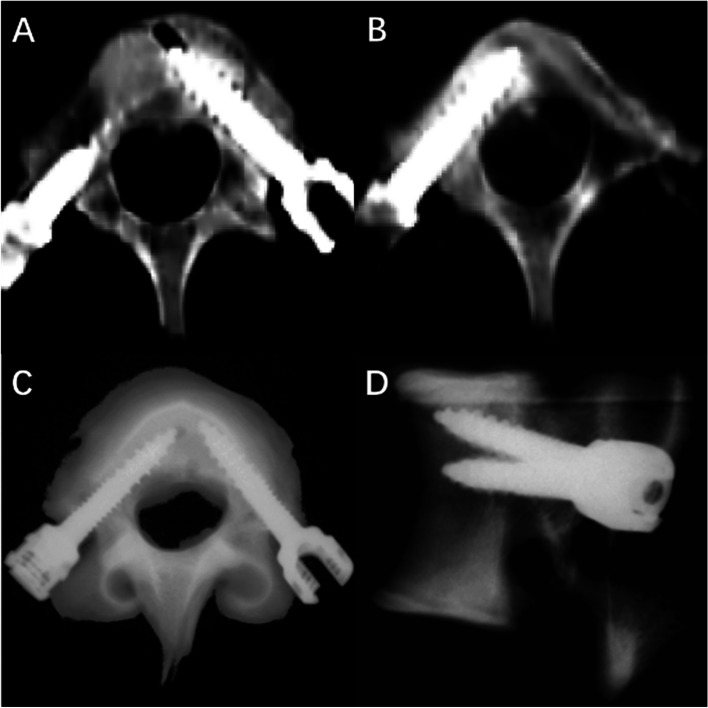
CT and X-ray detection of screw position. A and (B) are axial views of CT scans after pedicle screw implantation. A was the left screw view that is automatically planned, and (B) was the right screw view that is manually planned. C (D) was the X-ray examination after pedicle screw implantation. C was the bottom view of the vertebrae. The left screw of the image was the manually planned trajectory, and the right screw was the automatically planned trajectory. D was the lateral view of the vertebrae. The upper screw in the image was the manually planned trajectory, and the lower screw was the automatically planned trajectory. Neither screw resulted in pedicle perforation. The automatically planned trajectory has a smaller abduction angle and a larger caudal inclination angle than the manually planned trajectory
